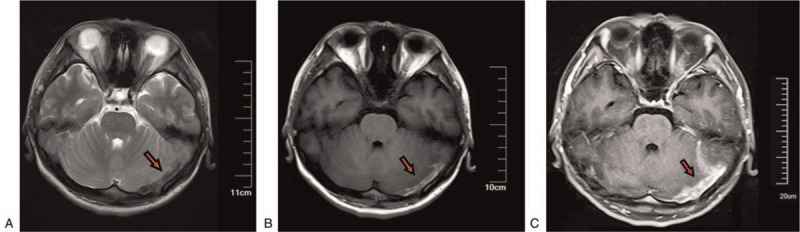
Images from pregnant women with transverse sinus thrombosis. A, T2-weighted image (T2WI) of MRI from a pregnant patient shows a clot in the left transverse sinus (red arrow). B, T1-weighted image (T1WI) of MRI from the same patient shows a clot in the left transverse sinus (red arrow). C, Contrast phase of MRI from the same patient shows a clot in the left transverse sinus (red arrow). MRI = magnetic resonance imaging.
